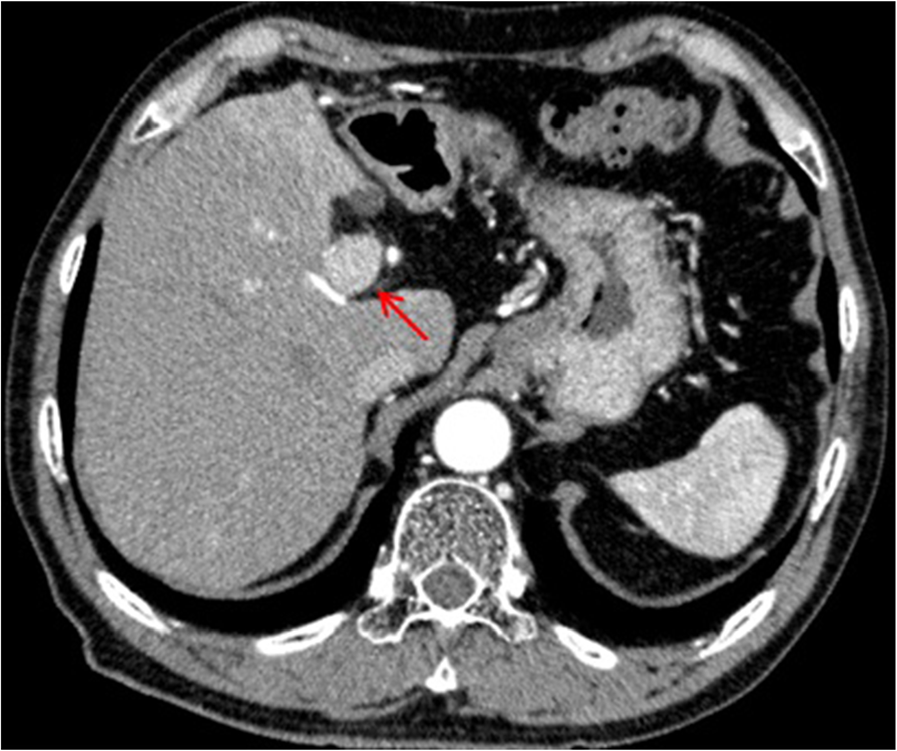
Computed tomography findings. There was a 30-mm contrast-enhanced space-occupying lesion in hepatic segment 4 at the early phase (*arrow*). The lesion presented with washout at the delay phase
